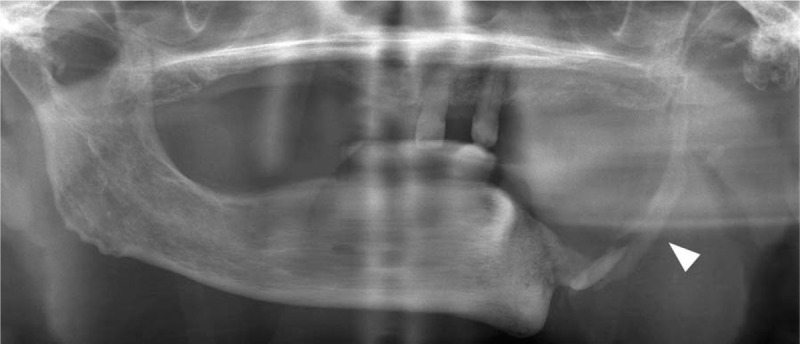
Follow-up orthopantomography 2 years after hemimandibulectomy revealed partial spontaneous regeneration of the left mandible (white arrow).
